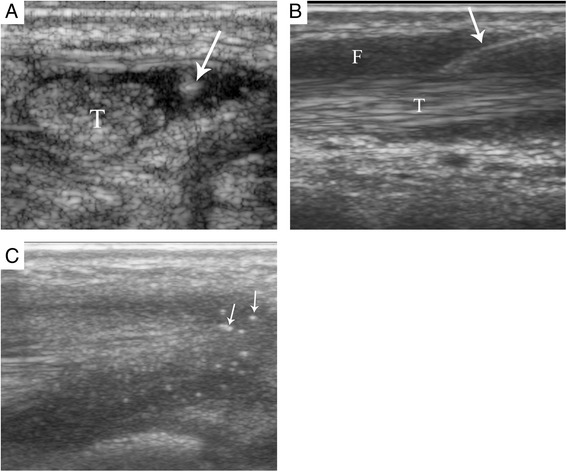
Sonographic images of tendon sheath injection approach. a An out of plane injection of the tibialis posterior tendon sheath of a 13 years 8 months old male. b An in plane injection of the tibialis posterior tendon sheath of a 9 years 11 months old female. *Arrows* point to the needle. c Steroid solution (*small arrows*) surrounding the tibialis posterior tendon of a 9 year 4 months old male after injection. T = Tendon, F = Peritendinous fluid
